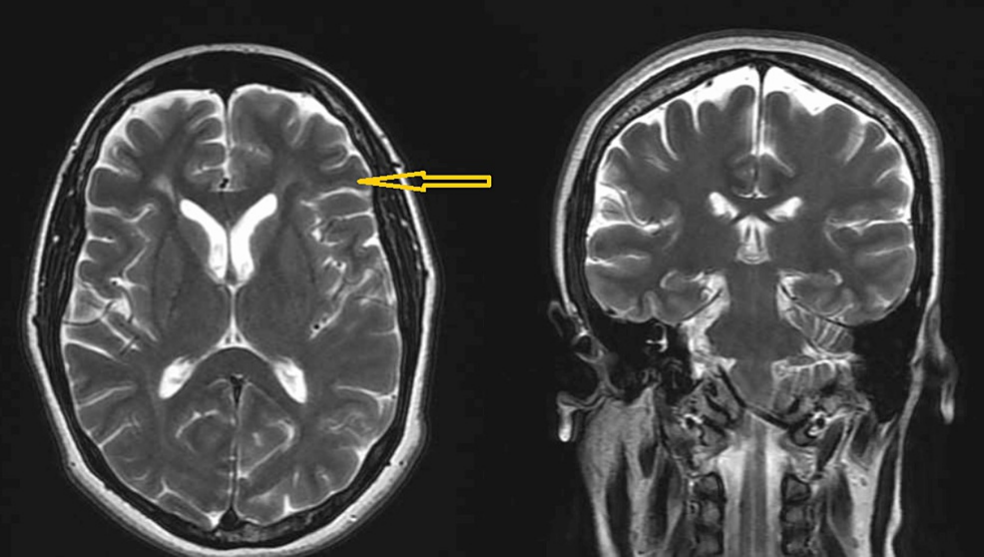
Axial and coronal T2W brain MR images showing normal findings
